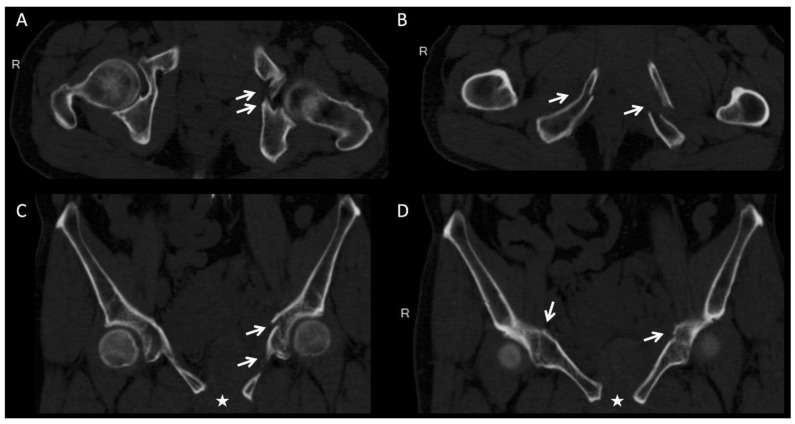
Computed tomography scan images showing bilateral superior and inferior pubic ramus fracture (arrows) combined with diastasis of pubic symphysis (asterisks). (A,B) Axial view and (C,D) coronal view.
